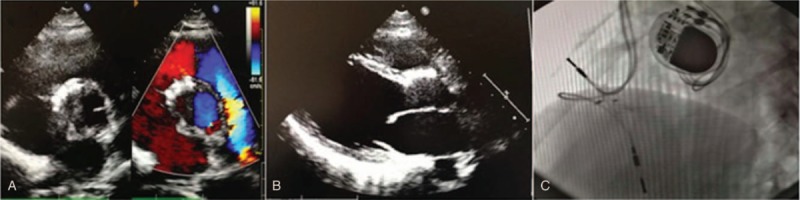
Findings on TTE and fluoroscopic evaluation over ten years post device closure. (A) parasternal short-axis view on TTE, (B) parasternal long-axis view on TTE, (C) fluoroscopic appearance of the device. TTE = Transthoracic echocardiography.
